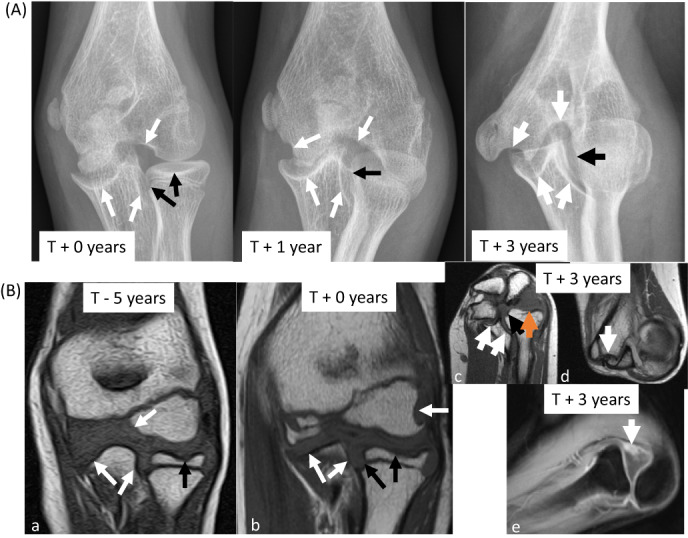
Images of left elbow. (*A*). Frontal radiographs of left elbow demonstrate multiple progressive erosions in the humerus and ulna (black arrows) as well as the radius (white arrows) with progressive deformity of the distal humerus and overgrowth of the subluxed radial head. (*B*) (a, b, c, d) T1 signal‐weighted MR images of left elbow demonstrate multiple progressive erosions in the humerus and ulna (blue arrows) as well as the radius (orange arrows) in the coronal plane (a, b). By 2020, the patient had a fixed flexion deformity that prevented acquisition in standard planes (c, d). (e) Post‐contrast T1 signal‐weighted image with fat saturation shows synovitis (arrow) and radiocapitellar subluxation. MR = magnetic resonance.
